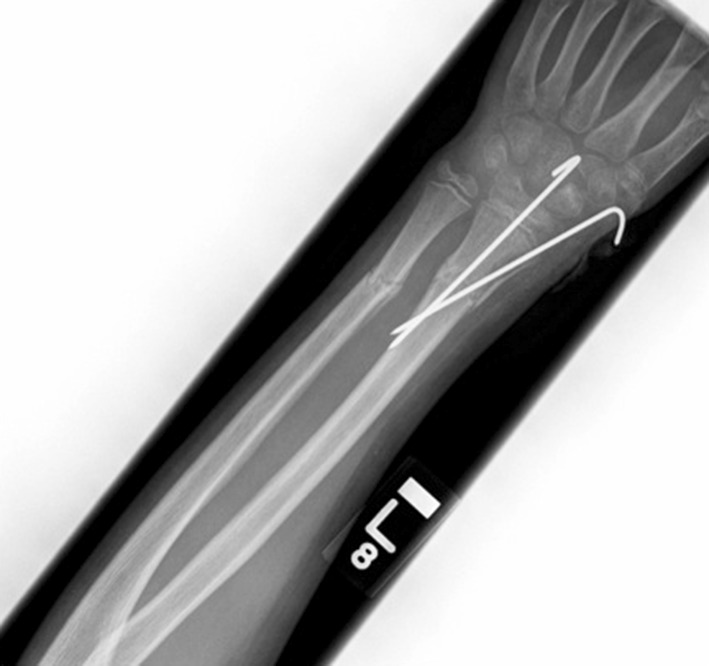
AP radiograph of the *left* forearm showing percutaneous K-wire fixation of a distal radius fracture
